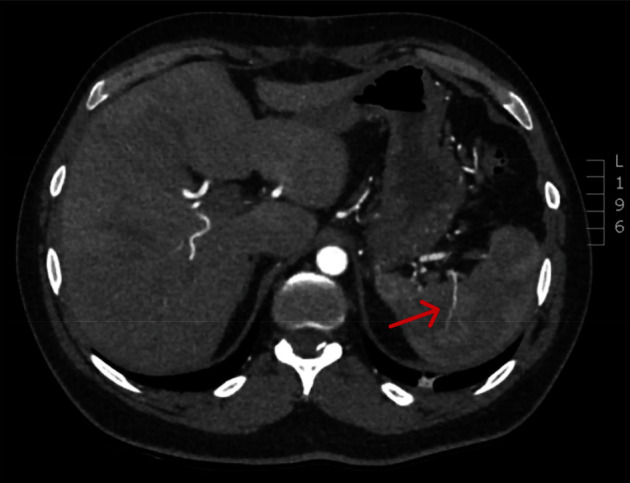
CT angiogram confirming adequate collateral perfusion of the spleen 3 weeks post-laparotomy.
